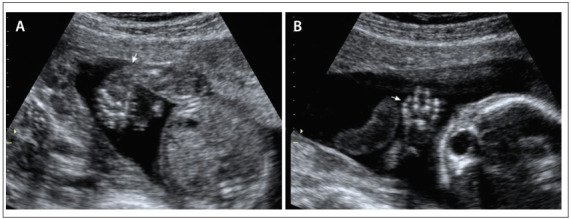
Prenatal ultrasonography findings of diastrophic dysplasia: (a) clubfoot; (b) fifth finger with clinodactyly.
